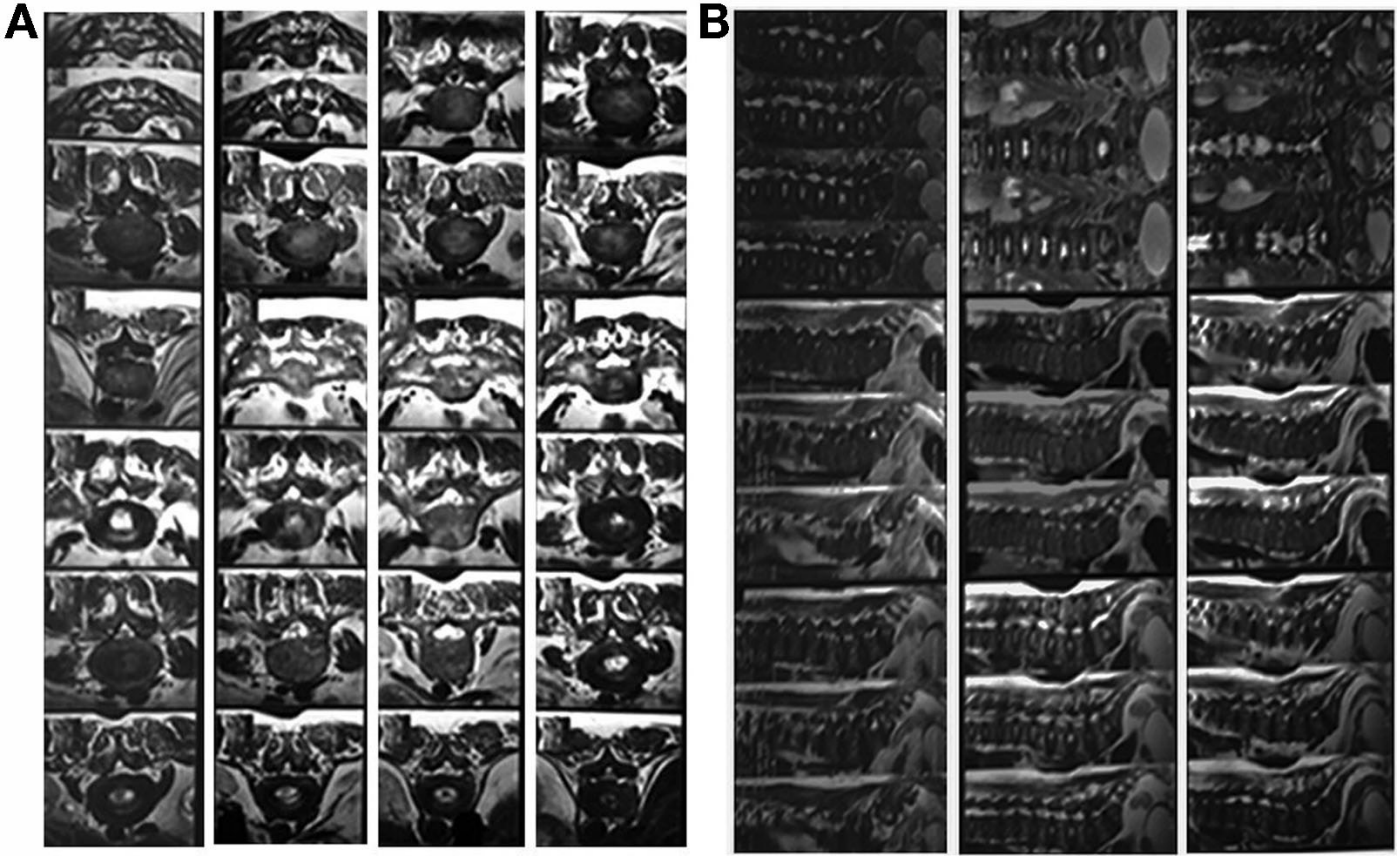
(A,B) MRI findings showing multiple myeloma-like changes.
